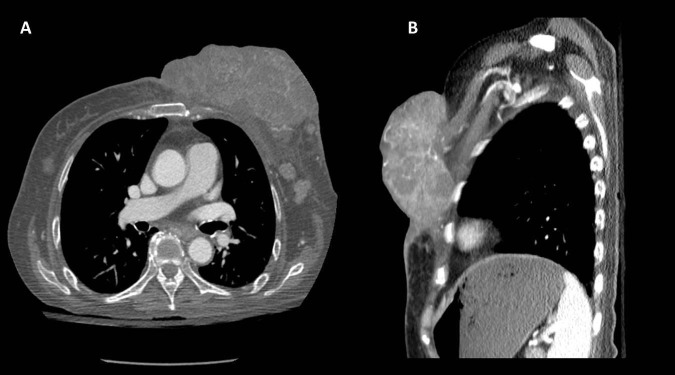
(A) Cross-sectional and (B) sagittal contrast-enhanced computed tomography (CT) imaging demonstrating the large left chest wall mass inseparable from the left pectoralis major muscle. Large mediastinal, axillary, and chest wall adenopathy can also be seen.
